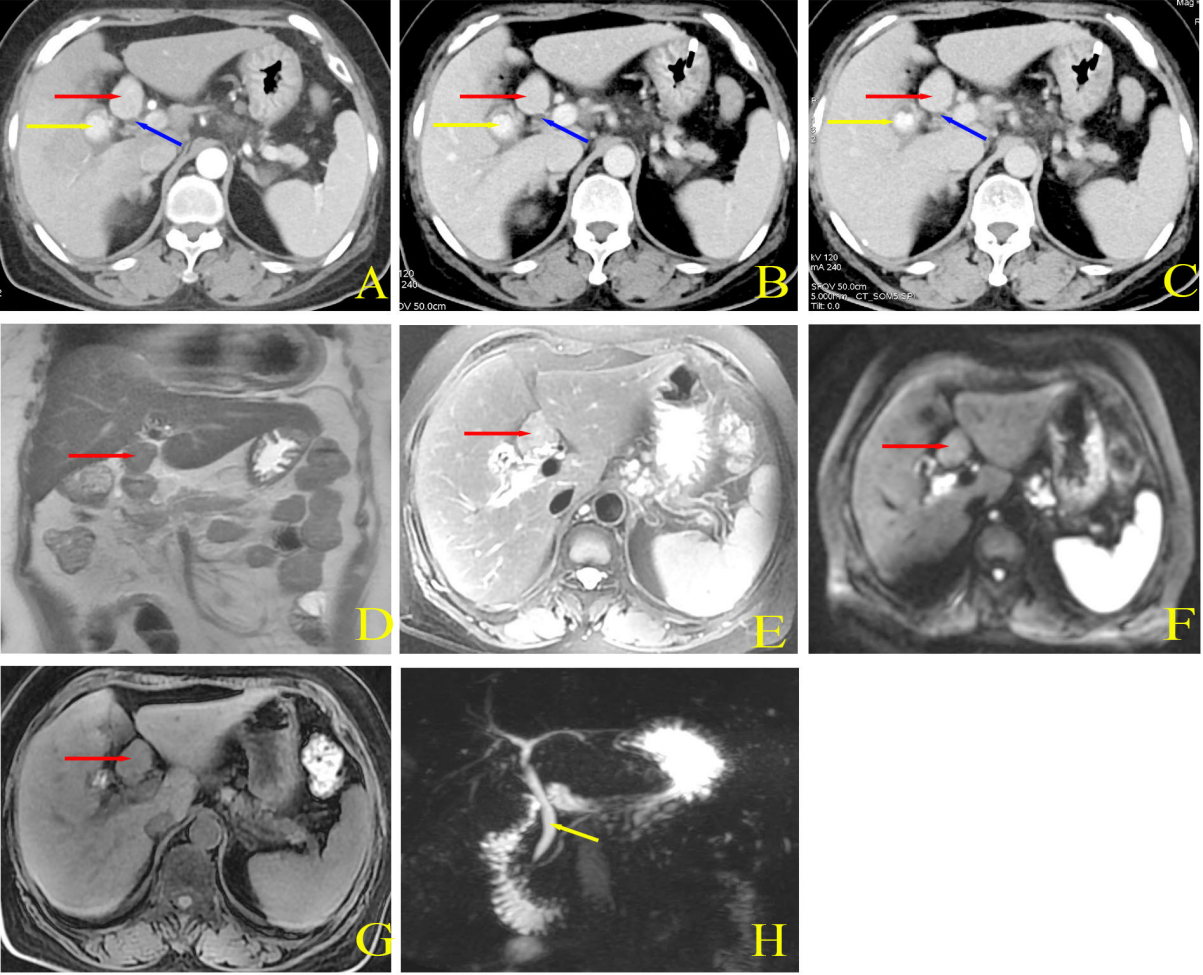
Preoperative abdominal CT imaging. (A) Arterial phase demonstrated ovoid mass with clear borders and markedly homogeneous enhancement. (B) Portal phase showed persistent enhancement. (C) Delayed phase indicated persistent enhancement, and the degree of enhancement was basically the same as that in the arterial phase. Red arrows indicated the mass, yellow arrows showed the gallbladder and intracystic stones, and blue arrows implied the common bile duct. Preoperative abdominal MRI and MRCP imaging. (D) Coronal T2WI indicated a mass located at the hepato-duodenal ligament with ovoid in shape and clear borders. The mass was also adjacent to the common bile duct. (E) T2WI showed isotropic signals. (F) DWI demonstrated isotropic plus slightly hyperintense signals. (G) T1WI also showed isotropic signals. (H) MRCP showed no dilatation of the bile ducts and no compression. Red arrow indicated the mass and yellow arrow indicated the bile duct.
